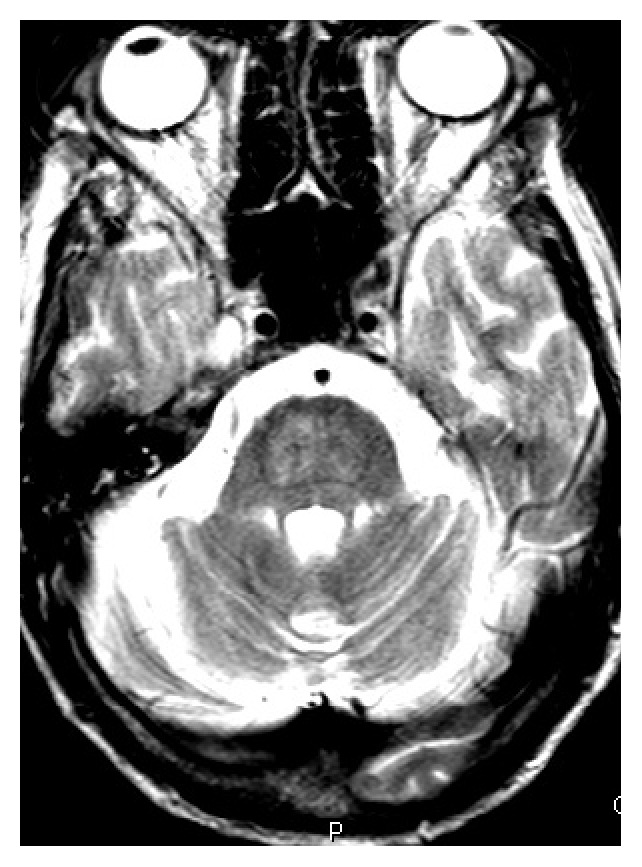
T2 magnetic resonance imaging demonstrating central pontine myelinolysis.
